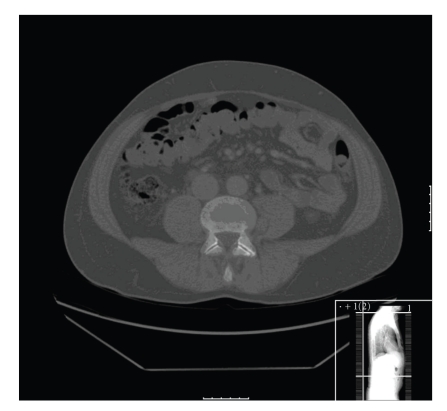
The CT image through L5 shows no significant abnormality on bone windows in contrast to the MRI scan at the equivalent level.
